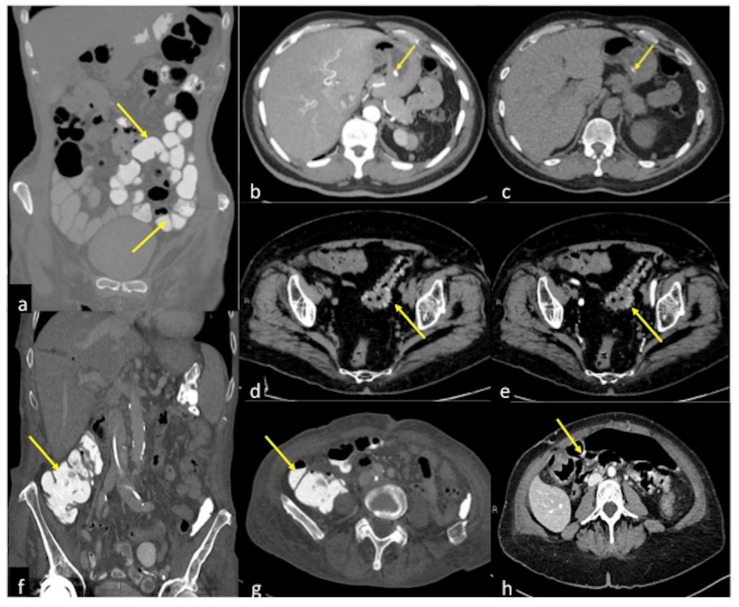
Pitfalls in the detection of acute gastrointestinal bleeding. (a) Positive contrast media administration (arrow): coronal CTA reconstruction of contrast media distended bowel loops (arrows) obscure any GIB source. (b,c) Endoluminal hyperdense ingested capsule: arterial and pre-contrast axial CTA image of an endoluminal hyperdense ingested capsule in patients with melaena. In the arterial phase with MIP post-processing (b), an endoluminal hyperdense inclusion is detected (arrow) in the gastric lumen; the pre-contrast phase (c) confirms the presence of the inclusion with analogous morphologic and densitometric characteristics (arrow). (d,e) Retention of contrast media in patients with diverticulosis and haematochezia: pre-contrast (d) and arterial (e) axial CTA images show unchanged hyperdense diverticula (d,e, arrow). (f,g) Hyperdense fecaloma: coronal (f) and axial (g) CTA image of hyperdense fecaloma (f,g, arrow) due to retained contrast media. (h) Cone-beam artefacts: axial arterial CTA image shows massive hyperdensities within the bowel lumen (arrow) due to cone-beam artefacts.
